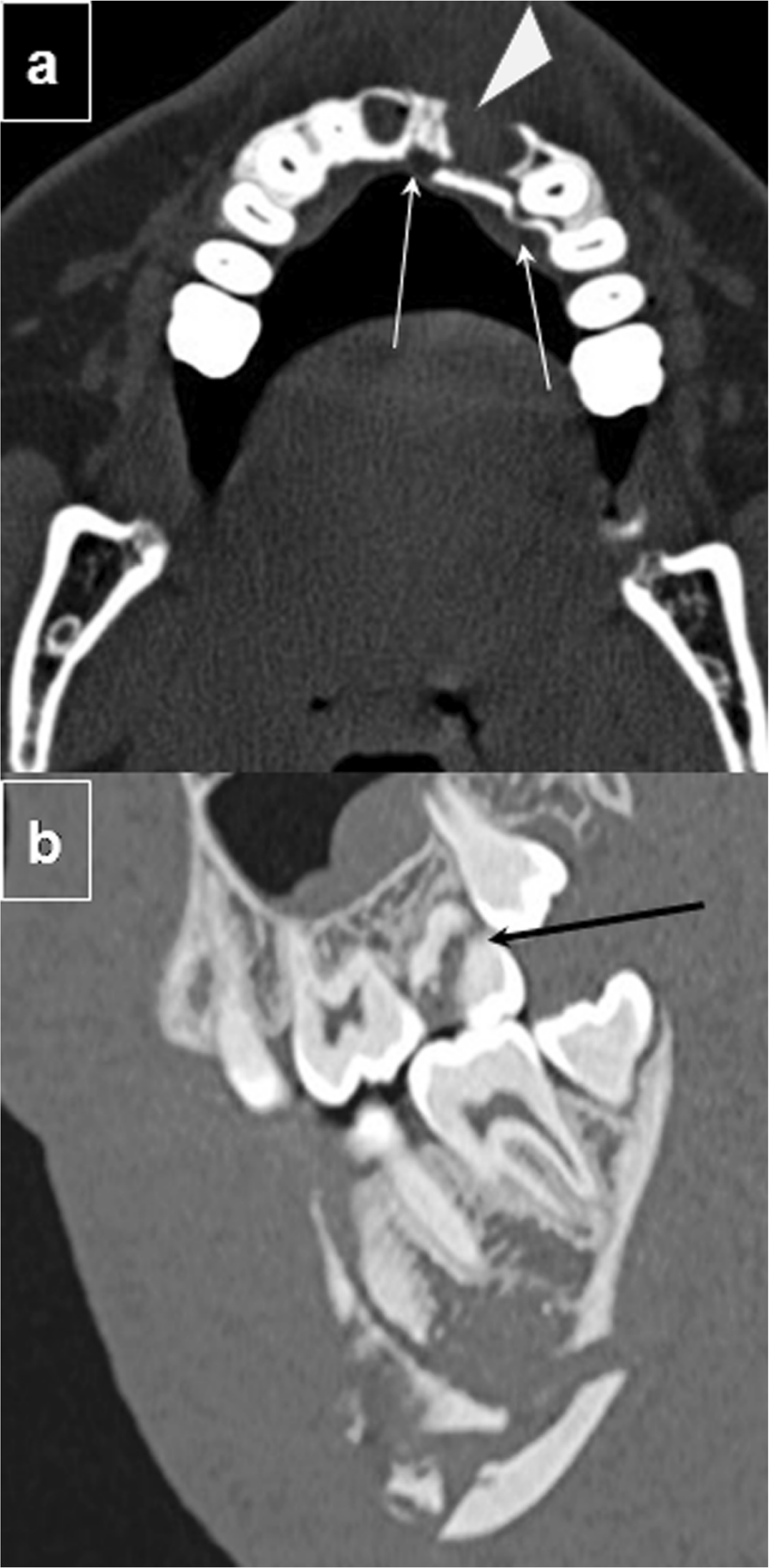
Dental complications. a Axial CT image shows a fracture of the upper alveolar ridge (thin white arrows) with avulsion of the left incisors (arrowhead). b Sagittal unenhanced CT image at the level of mandibular body demonstrates a molar crown fracture (black arrow) associated with a mandibular fracture
